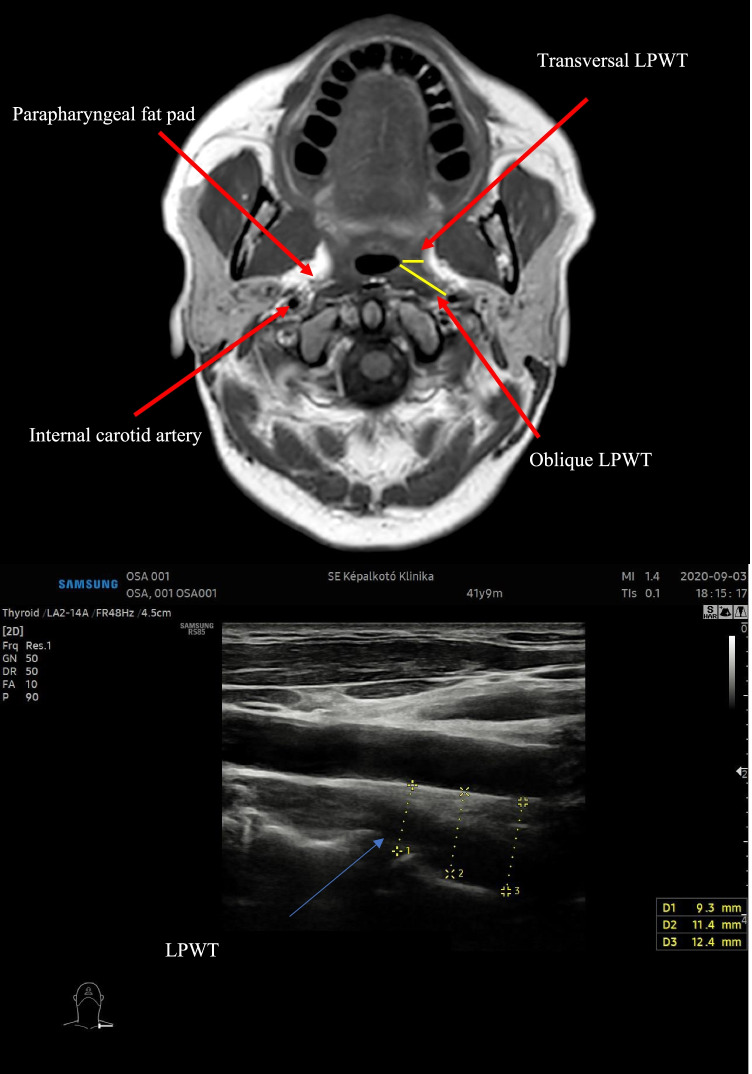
T1-weighed MR image (upper picture) of the LPW in the axial plane and US image (lower picture) in a gray-scale B mode. (Medical Imaging Centre of Semmelweis University). See the online version for coloured arrows
